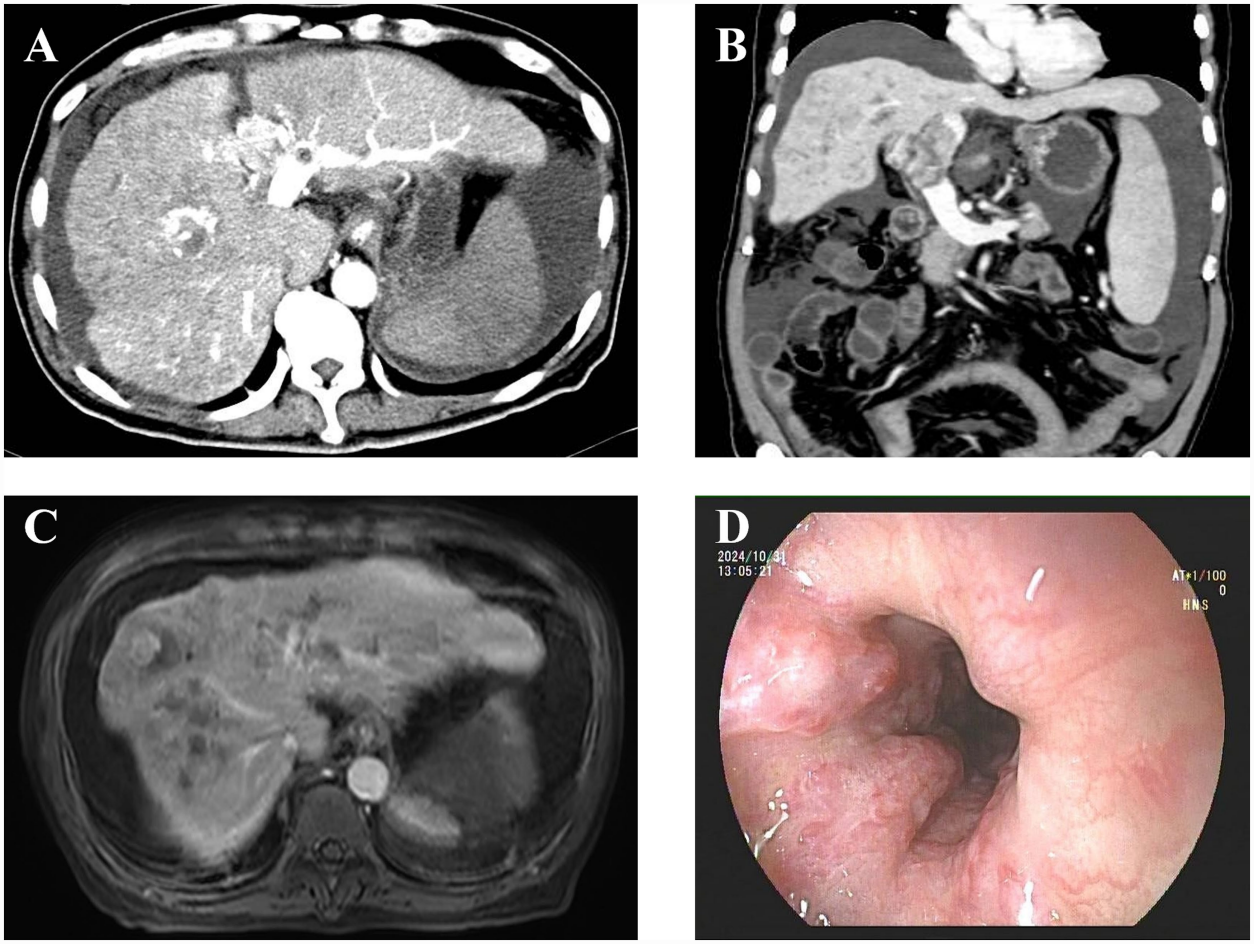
Preoperative imaging and endoscopic findings. (A) Axial CT showing tumor thrombus in intrahepatic portal branches (arrows). (B) Coronal CT demonstrating complete occlusion of the main portal vein (arrow). (C) MRI confirming HCC with extensive portal vein tumor thrombus (PVTT). (D) Endoscopy revealing severe esophagogastric varices.
